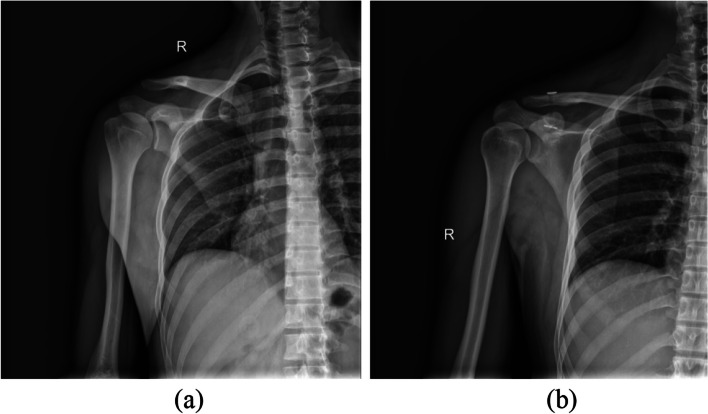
Basic information: male, right ACJ dislocation, (Rockwood type III) ,arthroscopic treatment with TightRope loop plate technique. a: the preoperative positive X-ray film showed elevation of distal clavicle and widening of ACJ gap and CCJ distance; b: the postoperative positive X-ray film showed good reduction of right ACJ
